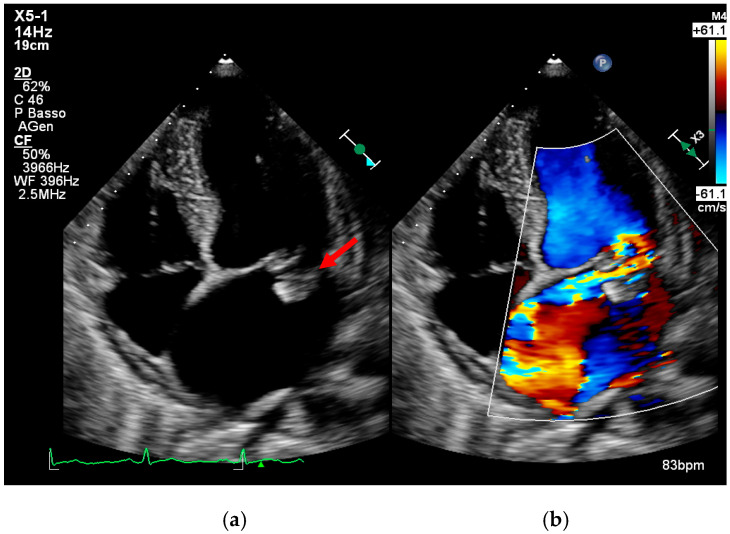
(a,b). Transthoracic echocardiogram showing mitral valve vegetation (arrow, a), causing perforation of the left posterior mitral valve leaflet and severe regurgitation (b). This is an original, de-identified clinical image from our institution. Patient consent for publication was obtained.
